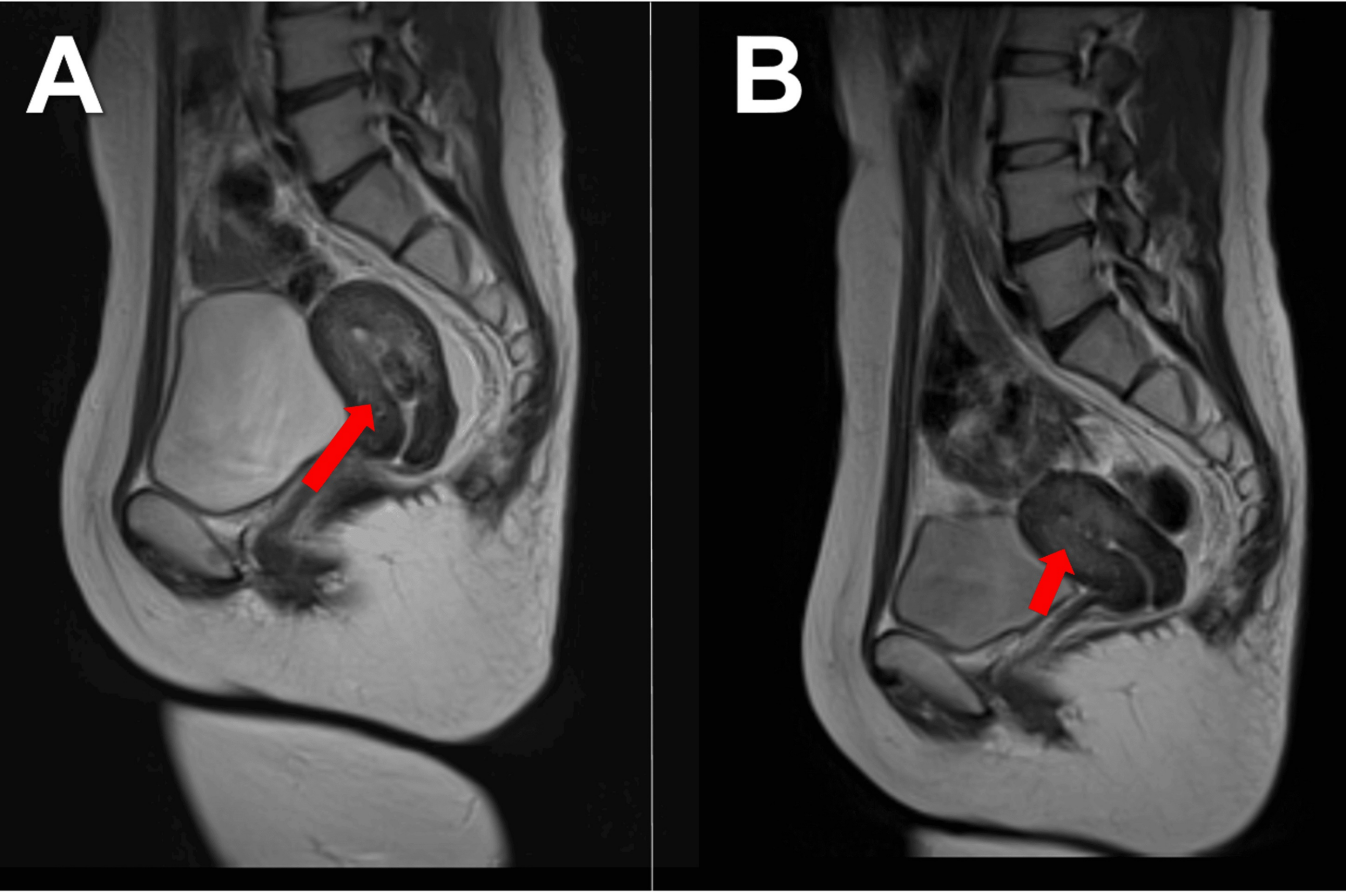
Serial MRI demonstrating progressive shrinkage of the prolapsed submucosal myoma after UAE and relugolix therapy (A) Sagittal pelvic MRI obtained approximately two months after UAE. The red arrow indicates the reduced submucosal myoma. (B) Sagittal pelvic MRI obtained approximately six months after UAE, immediately prior to TCR. The red arrow indicates further shrinkage of the submucosal myoma. UAE: uterine artery embolization; MRI: magnetic resonance imaging; TCR: transcervical resection
